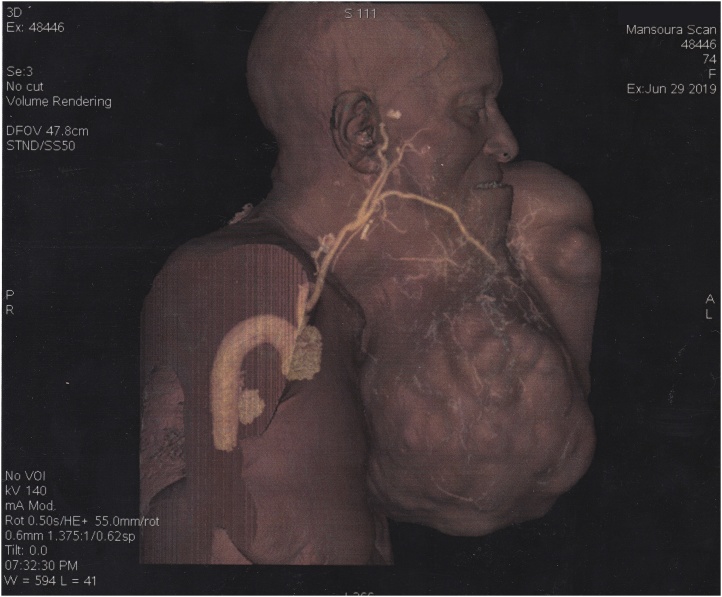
CT angiography (lateral view).
